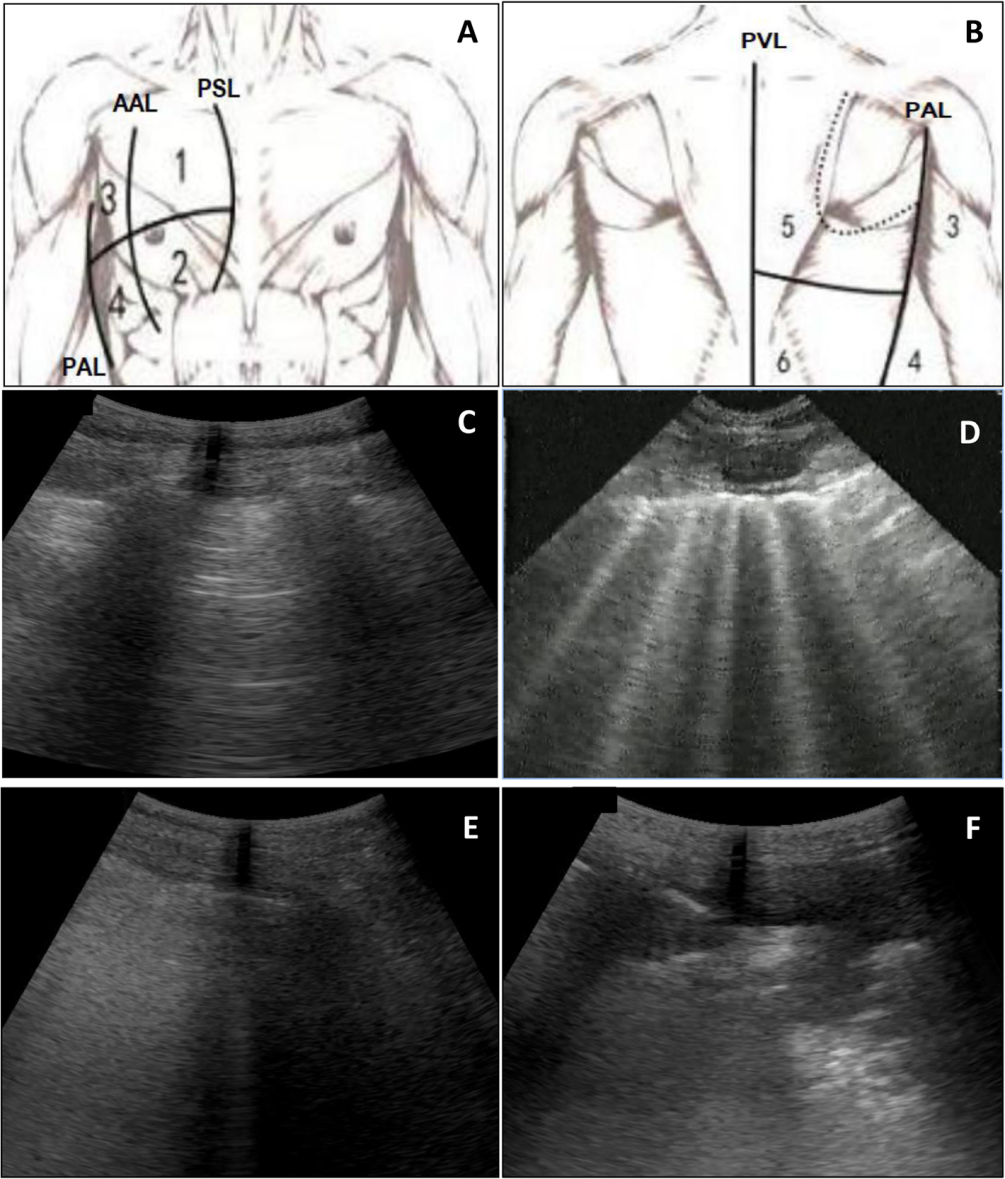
Ultrasound assessment of lung aeration score (LUS). Images a and b show 6 lung regions of the right lung; antero-superior (1), antero-inferior (2), latero-superior (3), latero-inferior (4), postero-superior (5), and posterior-inferior (6). Lung areas are delineated by parasternal (PSL), anterior axillary (AAL), posterior axillary (PAL), and paravertebral (PVL) lines. A numeric value was assigned to each lung area according to the most severe lung ultrasound finding. Images c, d, e, and f show typical images corresponding respectively to the degree of lung aeration loss. Image c shows normal lung image with presence of horizontal A lines (numeric value = 0); Image d shows the presence of multiple well-defined and spaced B1 lines issued from a small juxtapleural consolidation (numeric value = 1); image e shows multiple coalescent vertical B2 lines issued from the pleural line (numeric value = 2); image f shows the presence of tissue pattern containing either hyperechoic punctiform or linear images (numeric value = 3). LUS is calculated as the sum of the numeric values assigned to 12 lung zones ranging from 0 and 36
